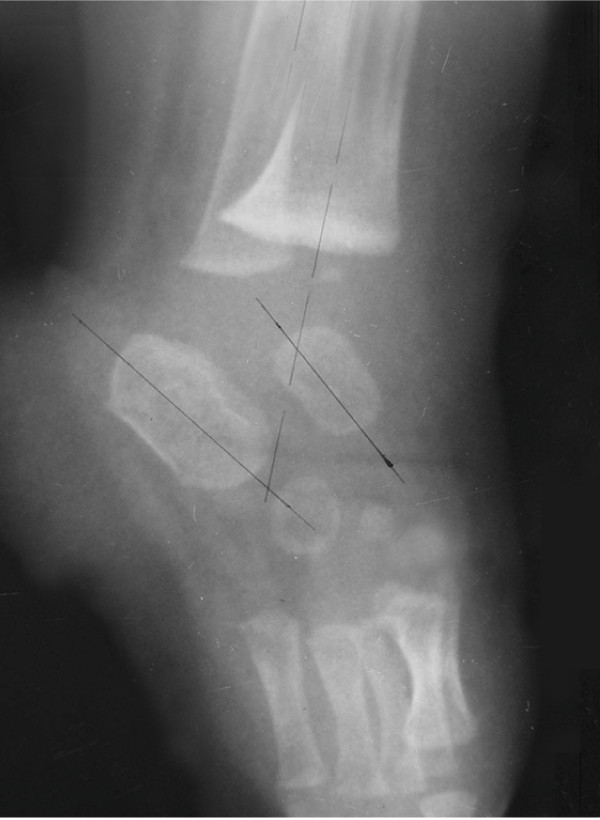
Preoperative plain lateral radiograph of the Rt. foot, with passive dorsiflexion of the foot, showing parallelism of the talus and calcaneus, severe equinus of the calcaneus and an acute tibio-calcaneal angle.
